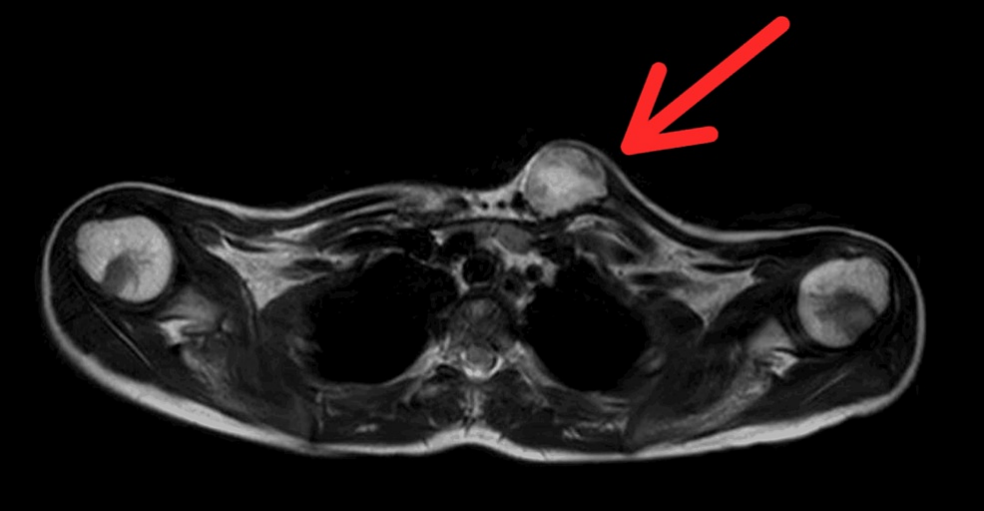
MRI showing the extent of the lesion
